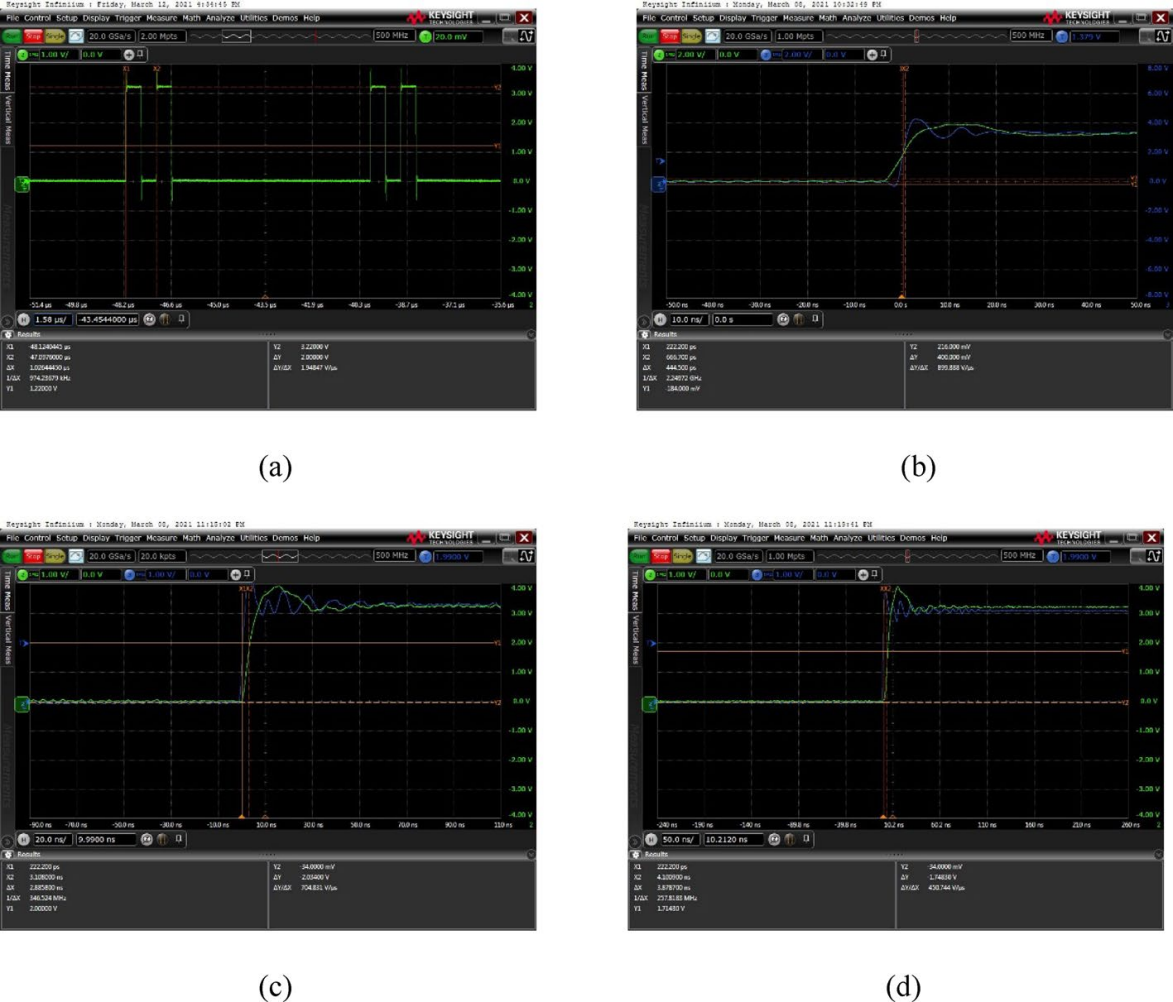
a Ultrasonic frequency and modulation waveform test results; b 1 and 2 channel delay resolution test results; c 1 and 3 channel delay resolution test results; d 1 and 4 channel delay Resolution test result
